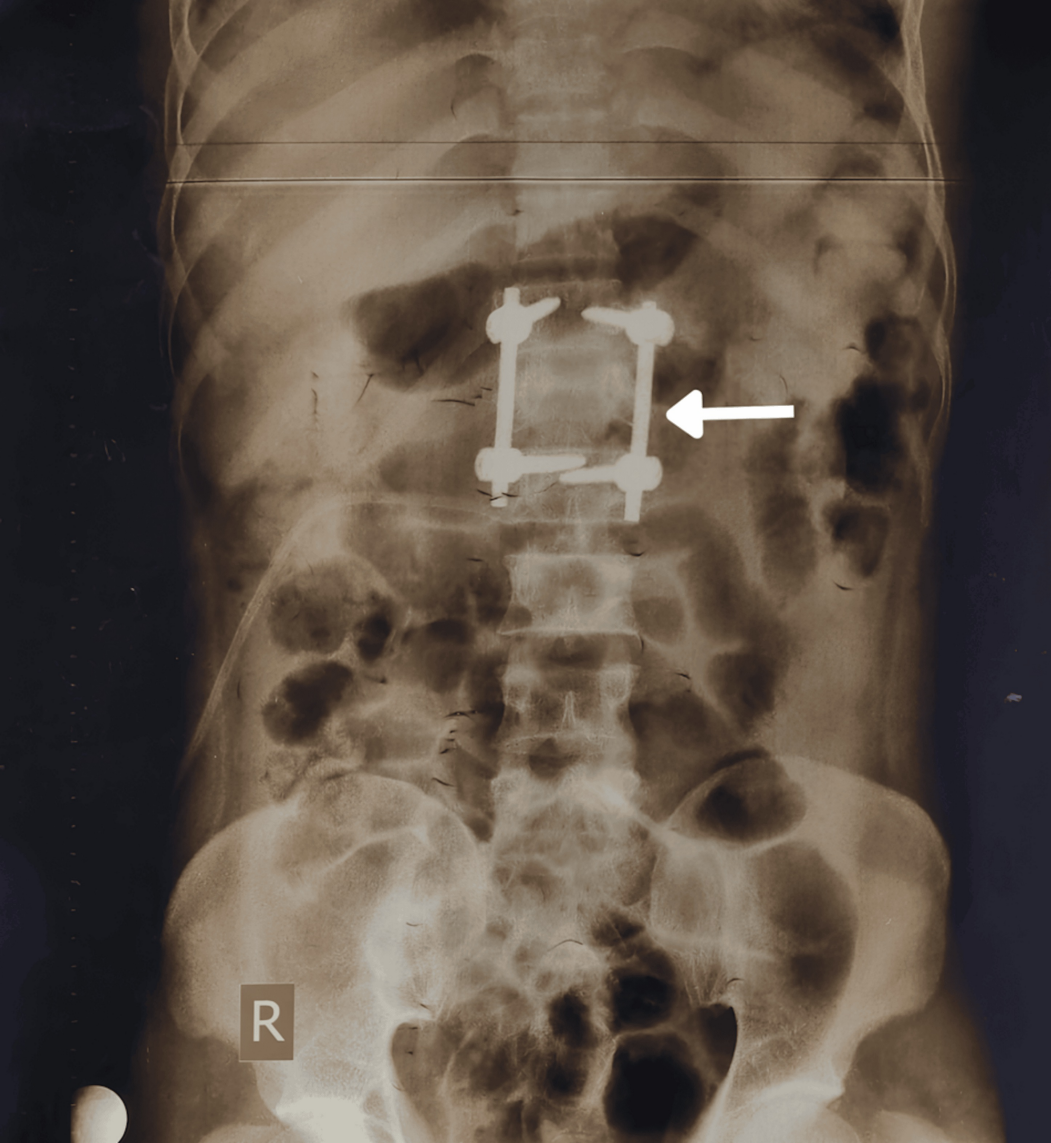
A radiograph obtained prior to his presentation with the burn injury showing spinal hardware (white arrow) indicative of previous spinal surgery.
